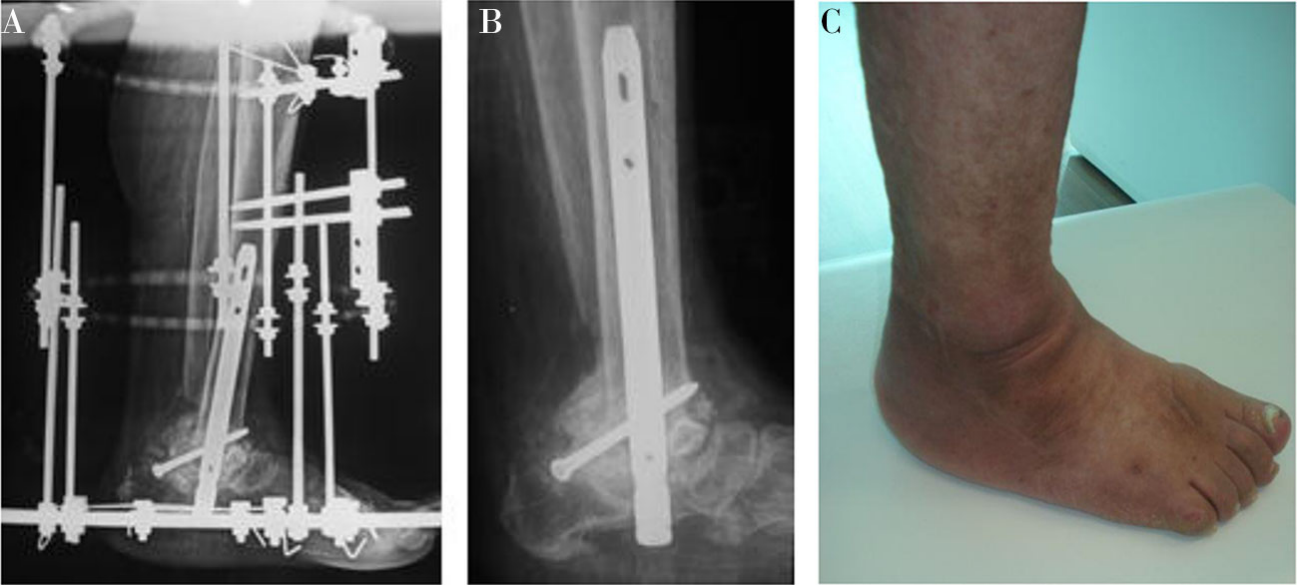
Postoperative lateral radiograph (a) showing the combined utilization of internal and external fixation, followed by external fixation removal (b) and clinical outcome 4 months after the surgery (c).
